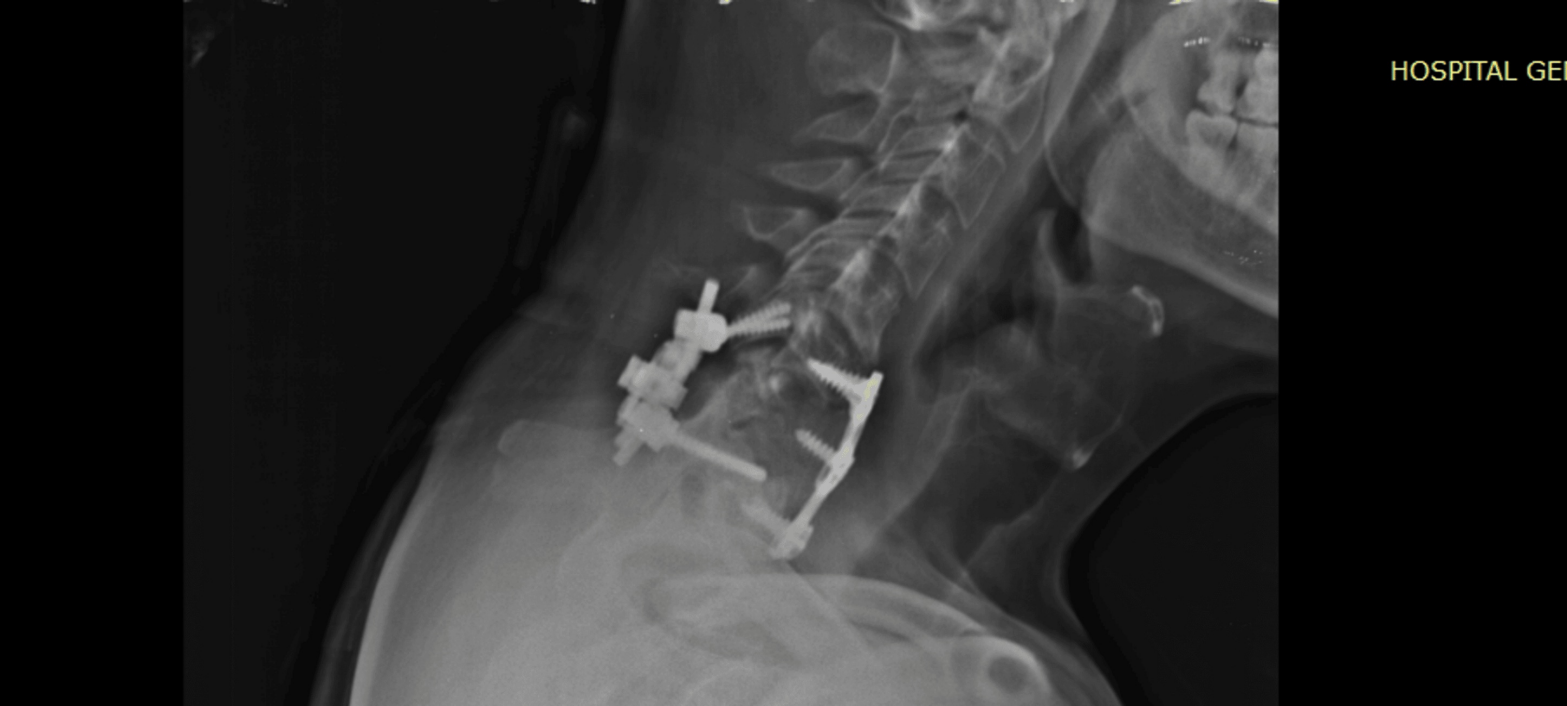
Case 4 post OP lateral X-ray of the cervical spine.
